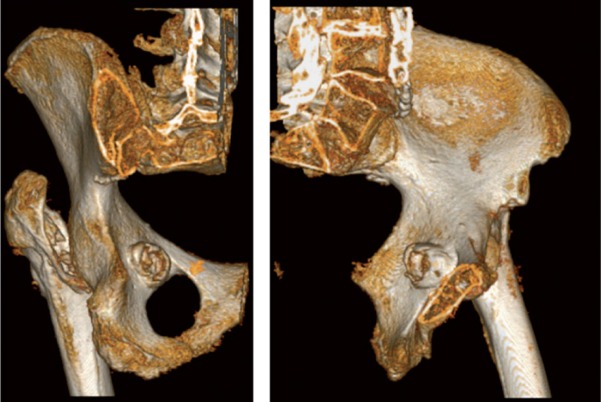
CT reconstruction (3D) of the entry hole through the pelvic bone, identifying the entry point of the screw to the abdomen before entering the rectum on its way to defecation.
